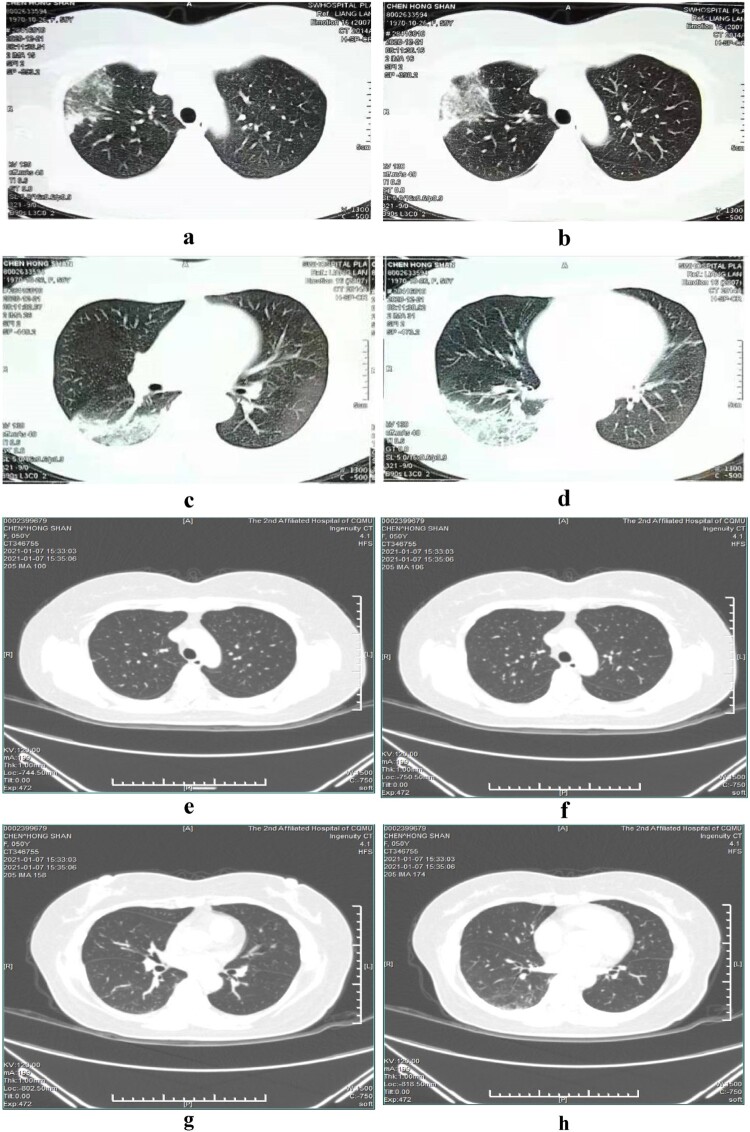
Serial chest computed tomography (CT) scans of the 40-year-old female with psittacosis pneumonia (the second patient). The initial CT scan (5 days after the onset) shows infiltrates, reticular shadows and consolidations with bronchograms in the right upper lobe (a, b) and right lower lobe (c, d). The follow-up CT scan (22 days after the onset) shows the area of infiltrates and consolidations in the right upper lobe (e, f) and right lower lobes (g, h) have both disappeared.
